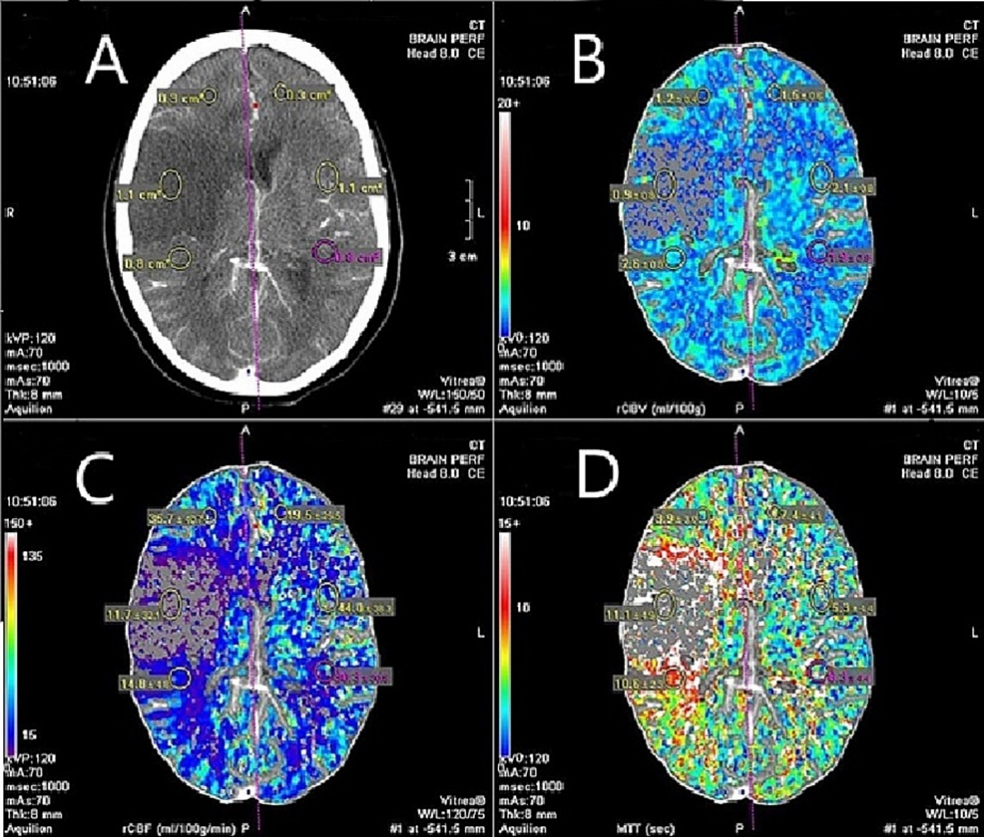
CT Perfusion images A. Non-contrast axial CT brain image showing right-sided acute ischemic infarction with regions of interest selected on the infarcted side and contralateral normal side. Assessment of perfusion parameters suggestive of acute ischemic infarction B. Decreased Cerebral Blood Volume. C. Decreased Cerebral Blood Flow. D. Increased Relative Mean Transit Time.
